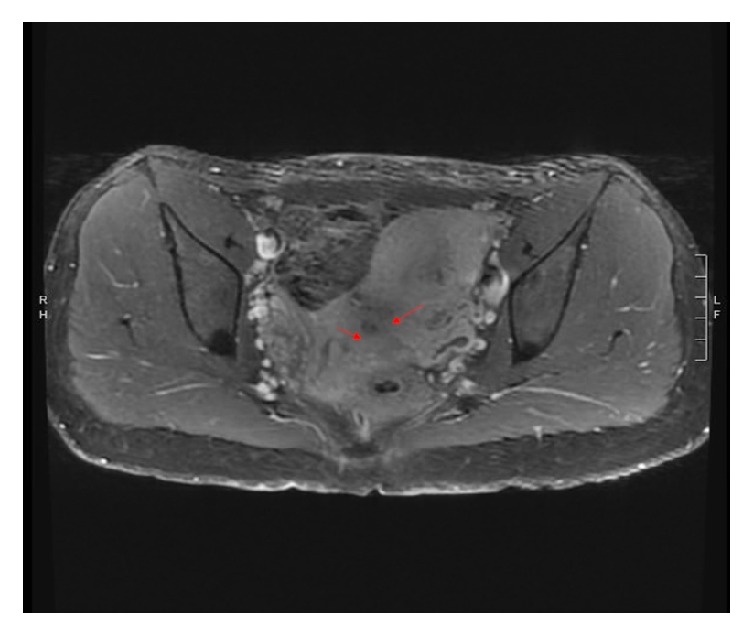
MRI demonstrating bicollis uterus.
